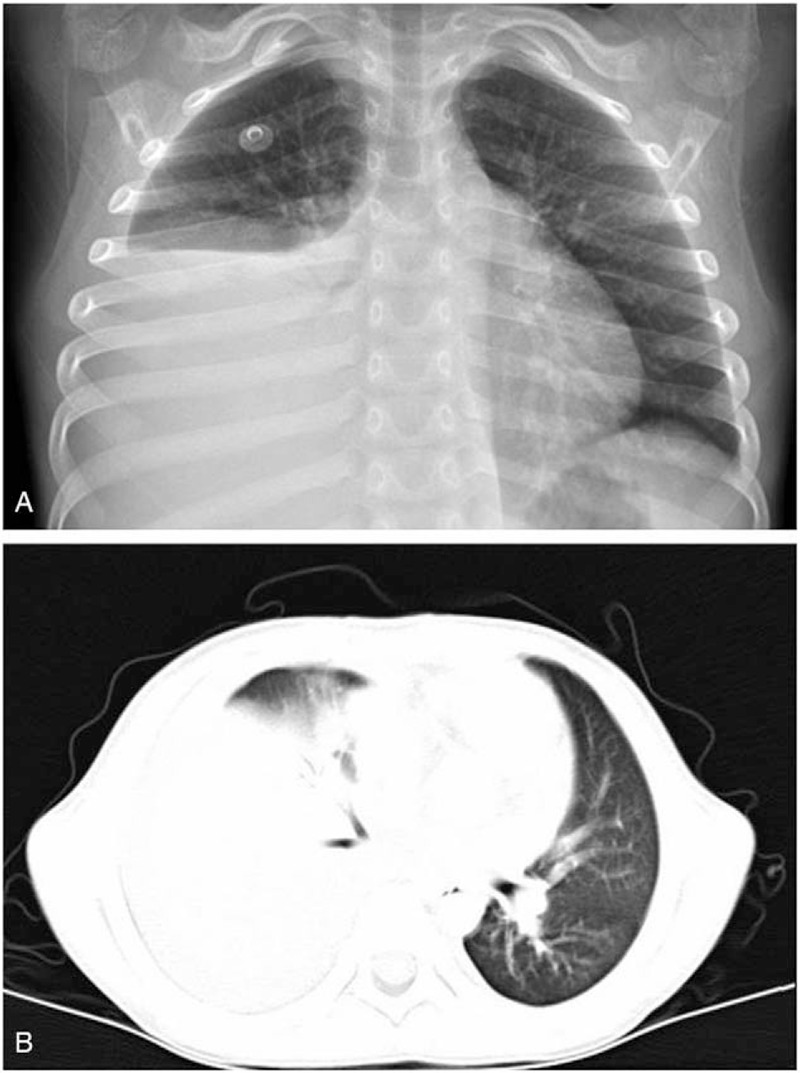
Chest X-ray and CT on admission. Chest X-ray and CT revealed a consolidation in the right lower lobe and massive pleural effusion. CT = computed tomography.
